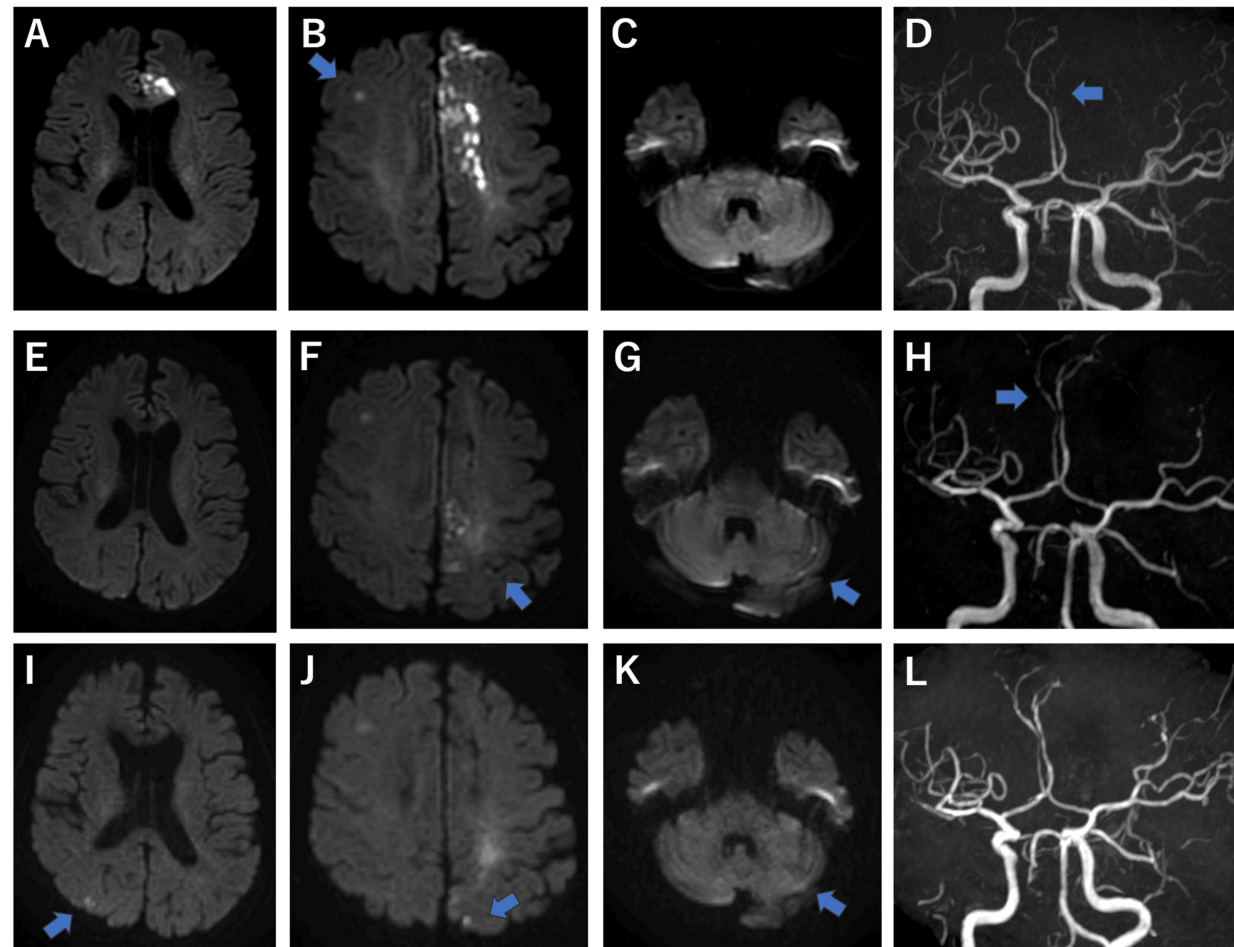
Time course of brain magnetic resonance imaging (MRI) Time course of brain magnetic resonance imaging: (A-D) During the first hospitalization (day 1). (E-H) During the second hospitalization (day 126). (I-L) During follow-up at the second hospitalization (day 139).
